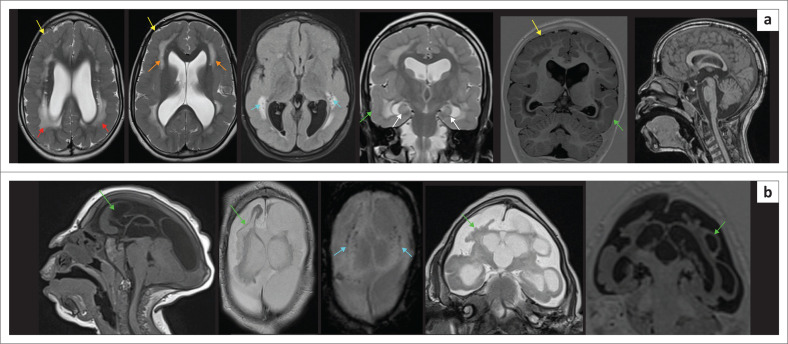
(a) Intrauterine cytomegalovirus *(CMV)* infection with a simplified gyral pattern (green arrows), polymicrogyria (yellow arrows), periventricular (red arrows) and subcortical (orange arrows) white matter hyperintensity with microcystic change (cyan arrows). Swollen hippocampi (white arrows) due to status epilepticus. Zellweger syndrome, Walker-Warburg syndrome and congenital lissencephaly type II are important differential considerations. (b) 7-month-old child with severe microcephaly. An MRI was performed to confirm suspected hypoxic-ischaemic brain injury for medicolegal evaluation. There is near complete destruction of the brain with periventricular calcification (cyan arrows). There is malformation of the cortex (green arrows) compatible with probable in utero *CMV* infection.
